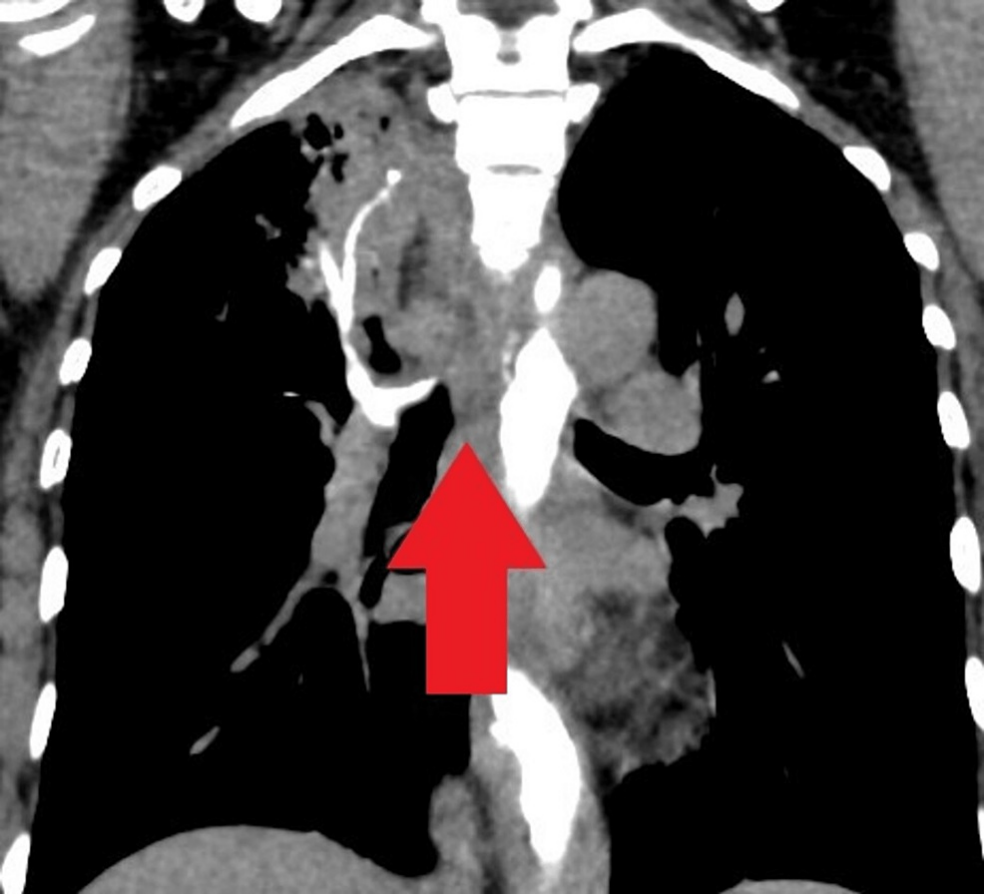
Computed tomography (CT) chest image. CT chest image red arrow pointing towards the extravasation of oral contrast into respiratory tract showing fistulous communication between esophagus and right bronchus.
